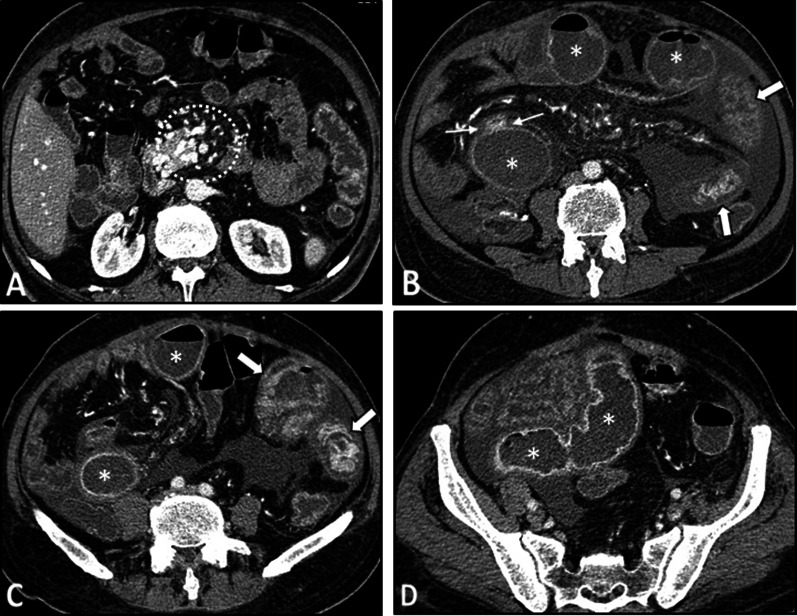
Bowel stricture with secondary bacterial overgrowth in a 44-year-old male with a history of primary antiphospholipid antibody syndrome. He presented with abdominal pain, fever, nausea, vomiting, and diarrhea without a history of previous surgery. CTE was obtained. Axial CT (A) shows evidence of chronic SMV thrombosis associated with multiple collateral veins in the mesenteric root (white dotted oval). CT images (B–D) demonstrate diffuse mural thickening at jejunal loops with submucosal edema and mucosal hyperenhancement (thick white arrows). There is an abrupt stricture in a segment of proximal ileum (thin white arrows) leading to upstream bowel dilatation (white asterisks) suggestive of partial obstruction with development of ascites. The clinical and imaging findings were suspicious for focal stricture secondary to an old vascular insult with secondary bacterial overgrowth in dilated jejunal and proximal ileal loops. The patient underwent antibiotic therapy, bowel rest, and conservative management resulting in improvement of signs/symptoms. He was discharged with an uneventful follow-up
